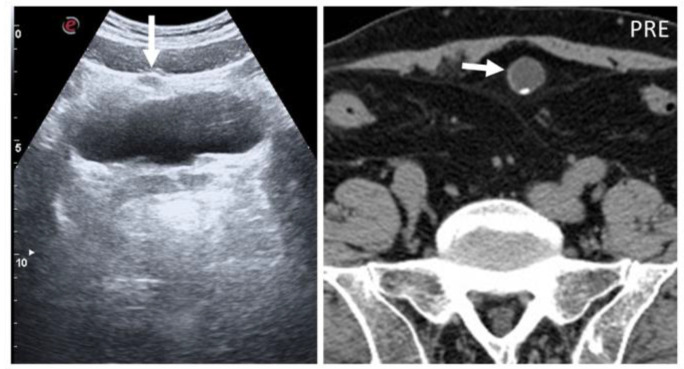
Urachal cysts are found along the superficial planes. On B-mode US, urachal anomalies appear as fluid-filled nodularity close to or in the context of the abdominal wall, incidentally found (arrow). As seen in axial, not-enhanced CT, small parietal calcification can also be seen (short arrow).
